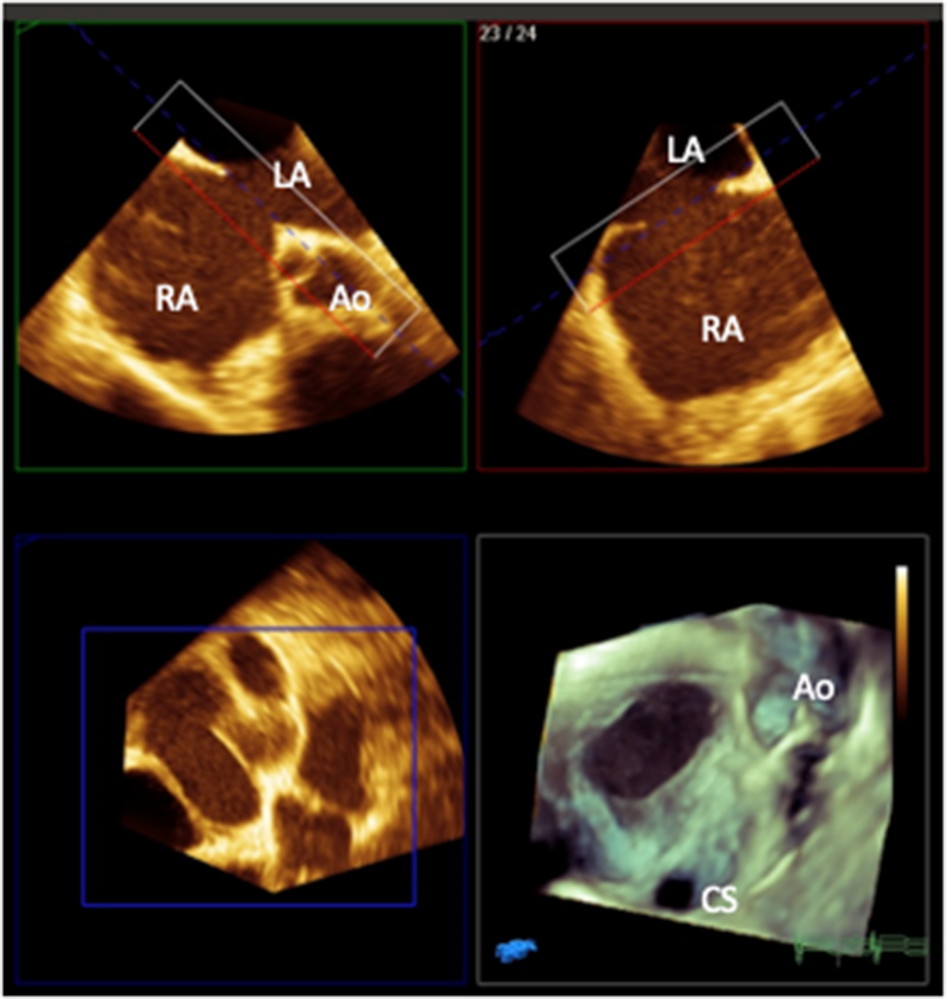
3D multiplanar reformat and rendered image (bottom right) of a transesophageal image of a secundum atrial septal defect with a deficient aortic rim as viewed from the right atrial aspect (Ao, aorta; CS, coronary sinus; RA, right atrium; LA, left atrium). Image courtesy of Alexandra Savis, Evelina London Children's Hospital.
